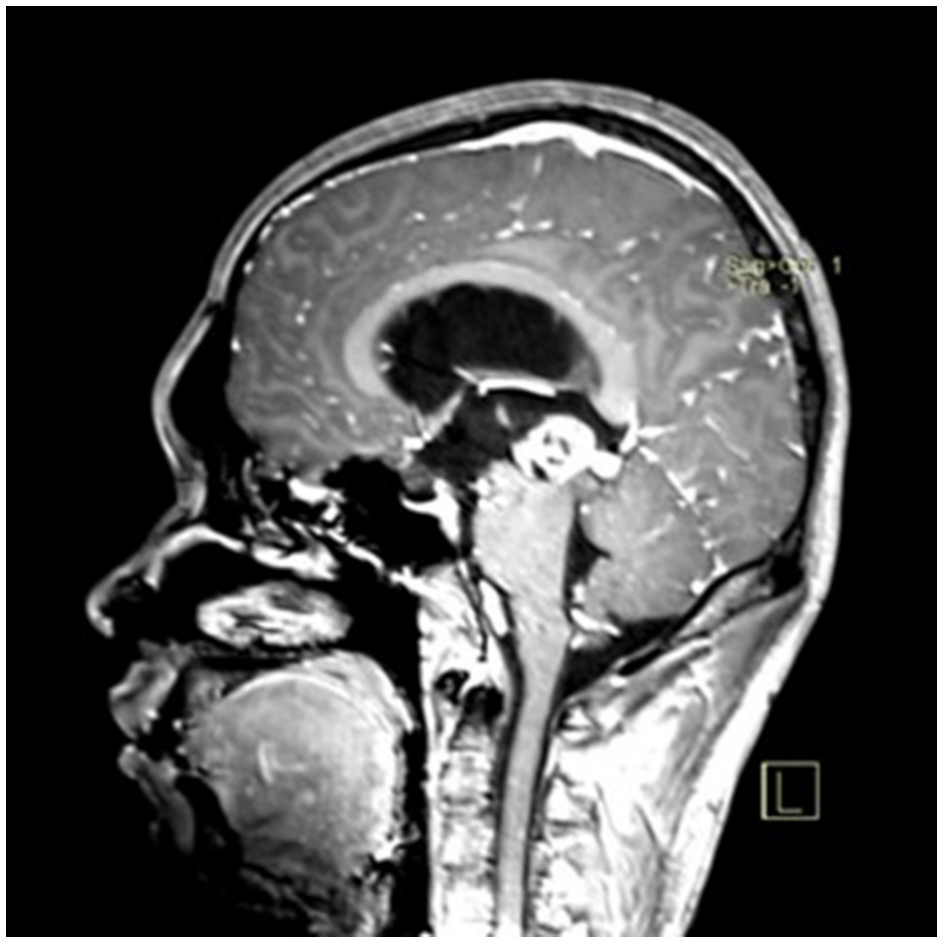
Preoperative contrast-enhanced MRI (sagittal view) showing the pineal region tumor.
